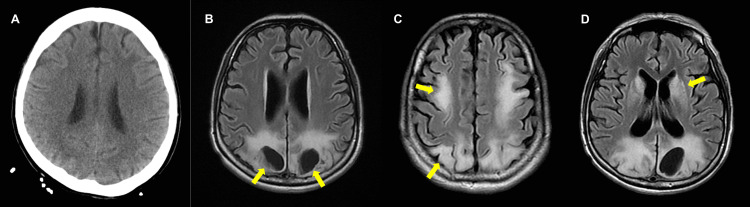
Computerized tomography of brain and T2-FLAIR brain magnetic resonance imaging Computerized tomography of brain performed 12 days after ROSC showing no remarkable findings (A). T2-FLAIR brain magnetic resonance imaging performed three months later revealing cystic encephalomalacia in bilateral parieto-occipital lobes (B), symmetrical T2-FLAIR hyperintense lesions, involving the bilateral fronto-parieto-occipital gray matter and white matter (C), basal ganglia (D), and splenium of the corpus callosum (not shown).
